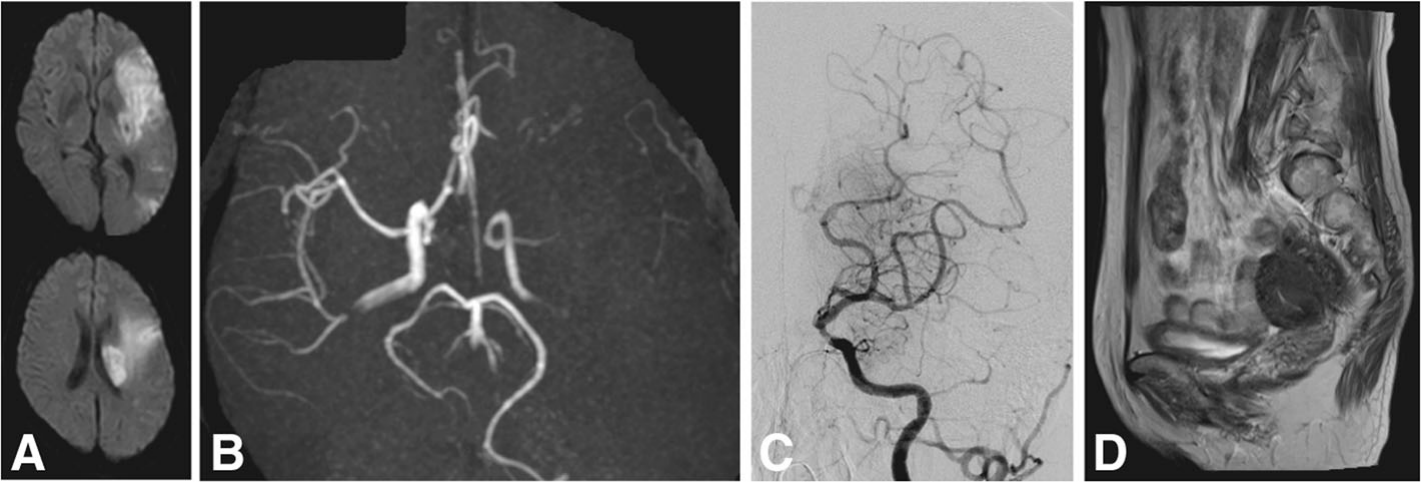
a Diffusion-weighted MRI revealed an infarct in the left middle cerebral artery territory. b Magnetic resonance angiography at a previous hospital showed left M1 occlusion. c Angiography revealed partial recanalization of the left middle cerebral artery. d Pelvic MRI revealed adenomyosis
